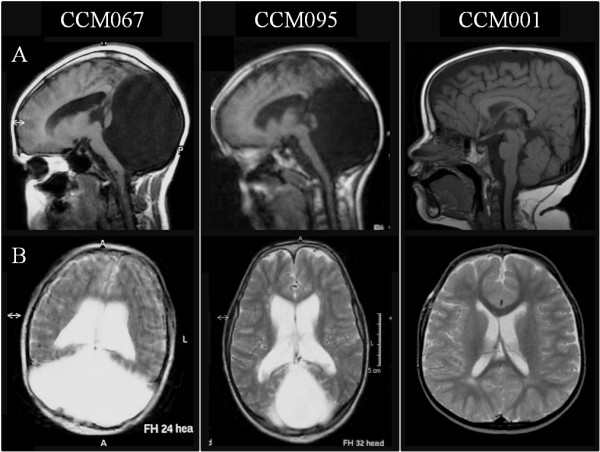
Brain MRIs. Brain imaging of patients CCM067, CCM095 (with Dandy-Walker malformation) and CCM001 (with normal cerebellum and posterior fossa). A: sagittal midline T1-weighted images. B: axial T2-weighted images.
